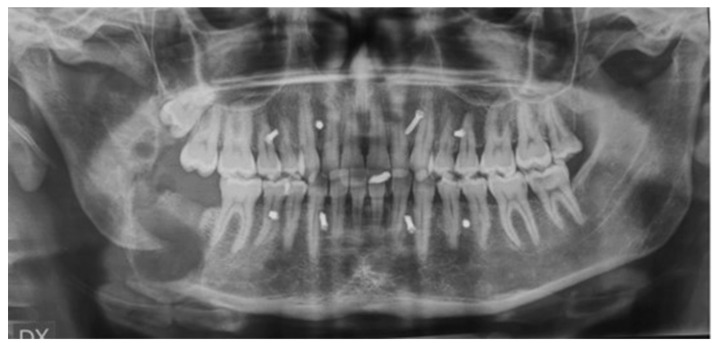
Pre-surgery panoramic X-ray showing a wide radiolucent lesion involving the body and ramus of the right mandible.
